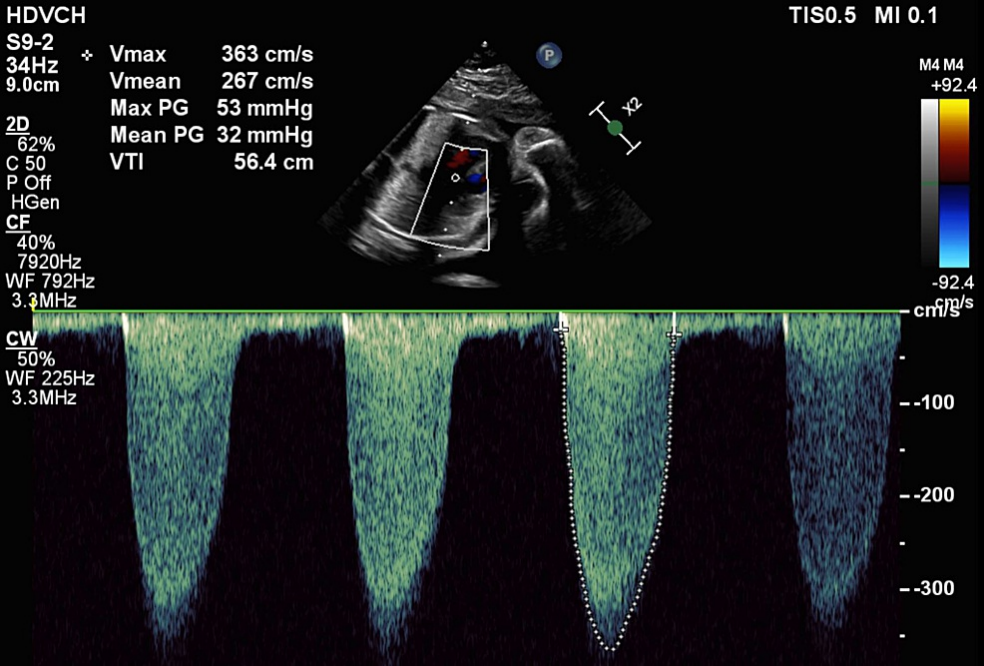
Doppler echocardiogram at 25 weeks of gestational age Pulsed wave Doppler demonstrating markedly increased pressures across the aortic valve with a maximum peak gradient of 53 mmHg and a mean of 32 mmHg (peak velocity: 3.6 m/s)
